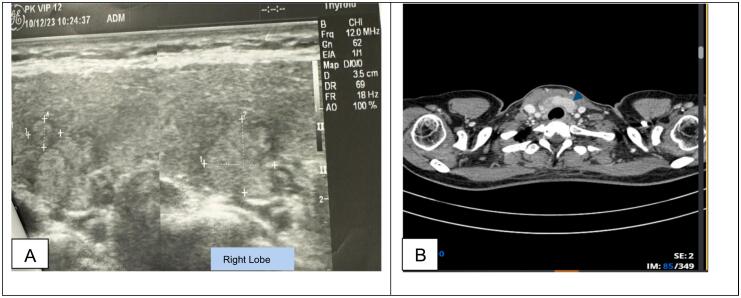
Ultrasound and CECT images of the patent: (A) The ultrasound revealed a large solid mass (38x25mm) in the anterior cervical region, with an ill-defined margin to the right thyroid, classified as a TI-RADS 5 thyroid nodule due to the extension of the lesion with some suspicious cervical lymph nodes, which the largest lymph node was 8x5mm in size. (B). On the non-contrast CT-Scanner images, a heterogenous hyperenhancement solid mass was detected at the same region, above the superior border of the manubrium sternum bone, anterior to the trachea, brachiocephalic vein, caudal to the thyroid, and invaded to the right thyroid lobe, with mild infiltration to the surrounding fat tissue. Some suspicious hyper-enhanced lymph nodes (level VI) were detected; the largest one was 14 × 8 mm in size.
